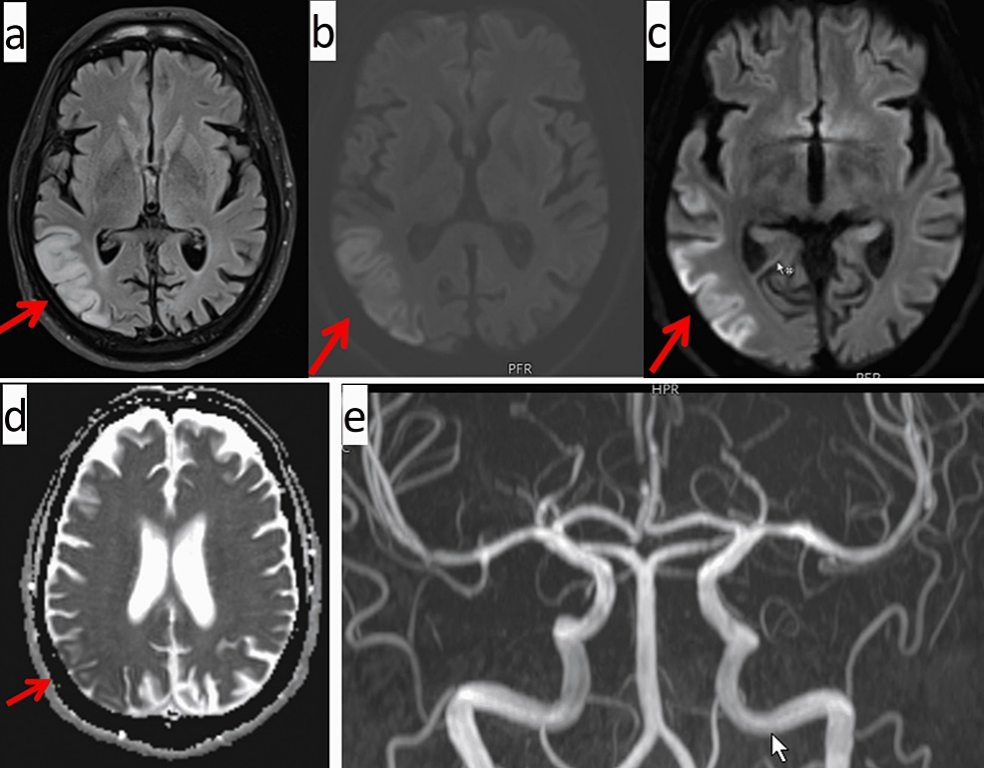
Cerebral MRI on hospital day 2 showing a T-hyperintense lesion in the right occipitotemporal (a), which was hyperintense on DWI (b, c), and slightly hypointense on ADC (d). Additionally, there was a slightly T2- and DWI hyperintense lesion in the left posterior borderzone. MRA was normal (e).
